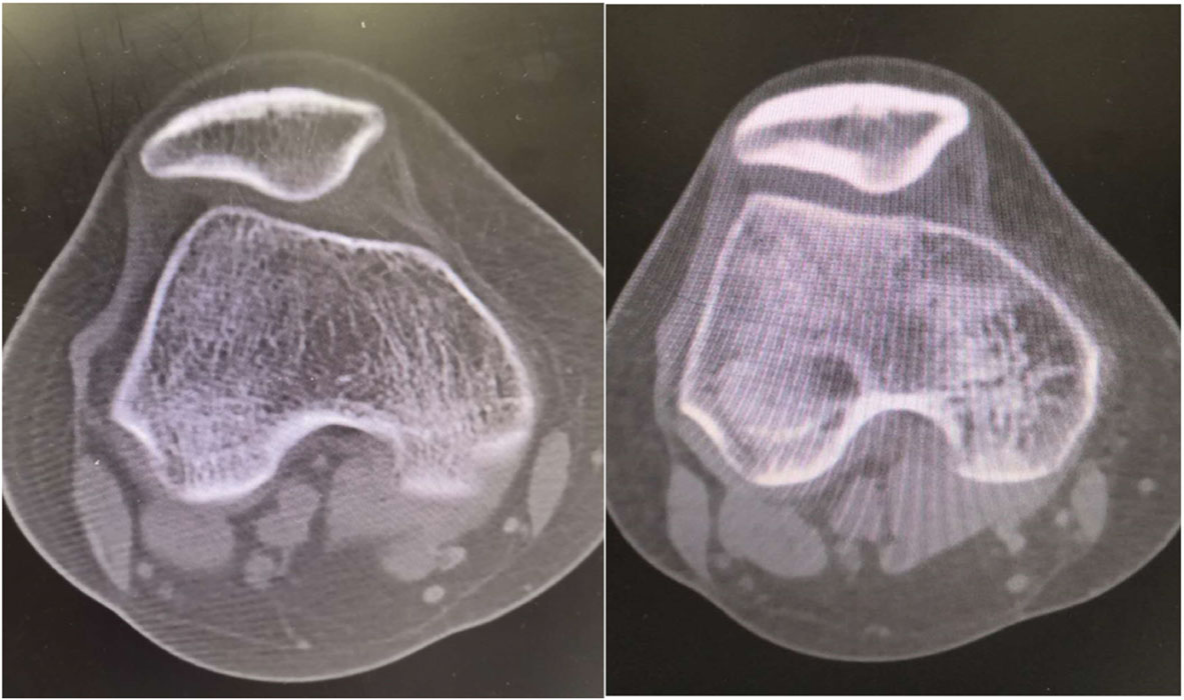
Preoperative CT images of a 27-year-old patient (left) and ipsilateral images on the second day after surgery (right)
